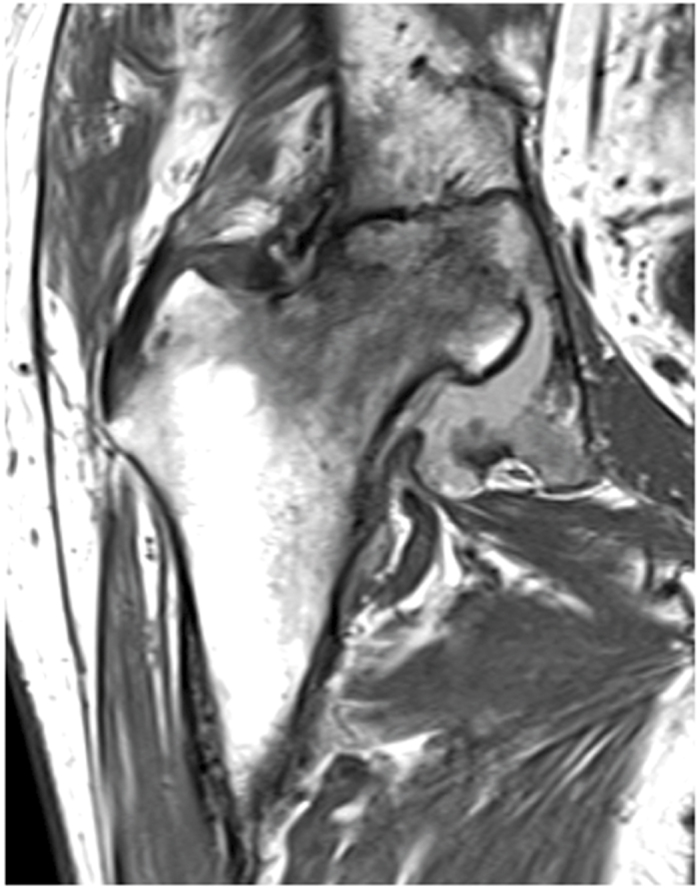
T1-weighted magnetic resonance imaging.
